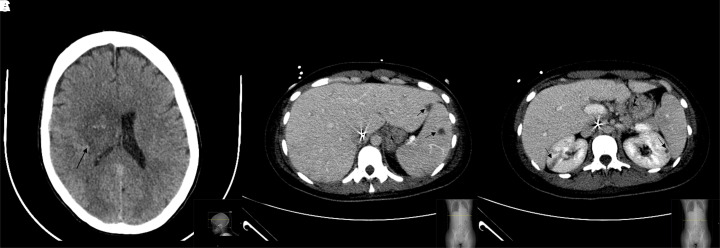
(*A*) Brain CT scan showing deep ischaemic lesion in temporal right hemisphere, with an increased density in the middle (black arrow), compatible with petechial non-confluent haemorrhagic transformation, and mass effect; (*B*) hepatomegaly with small hypodense nodules in the liver and spleen (black arrow), compatible with small abscesses; and (*C*) bilateral renal wedge-shaped hypodense lesions (black arrow), corresponding to small areas of infarction.
